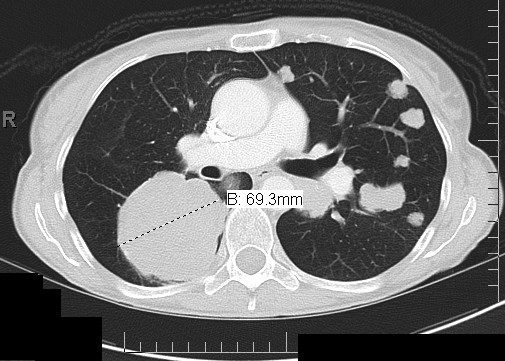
CT scan demonstrating progression of disease after first line palliative chemotherapy with the CAP regimen.
